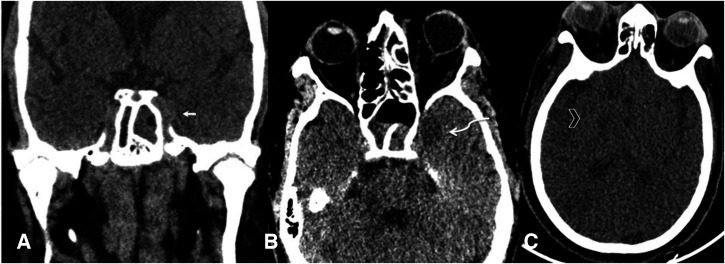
Coronal (A) and axial (B, C) computed tomographic sections showing intracranial manifestations of mucor. Cavernous sinus involvement presents as bulky cavernous sinus with loss of normal contour (arrow, A). The adjacent temporal lobe in the same patient also shows ill-defined hypodensity, suggesting cerebritis/abscess formation (curved arrow, B). Axial noncontrast computed tomography in a different patient (C) shows hypodensity in the right basal ganglia (arrowhead), the internal capsule, and the temporo-occipital region, suggesting infarcts.
